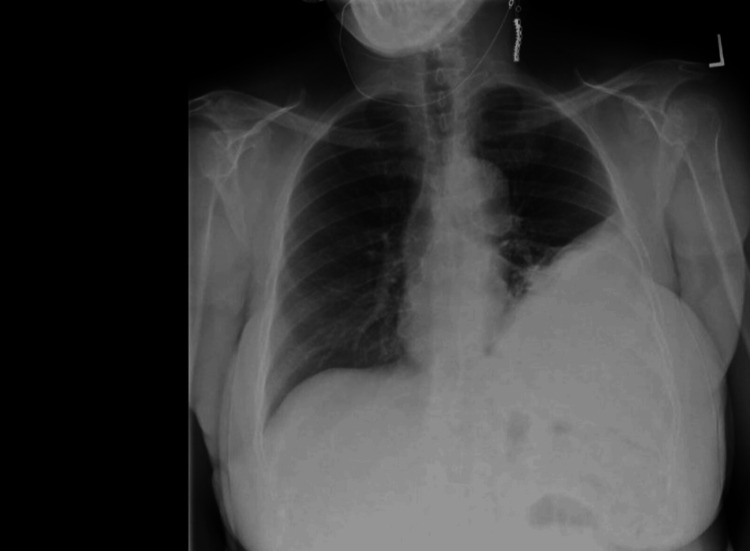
Initial Screening Chest Radiograph Showing Left-Sided Pleural Effusion
